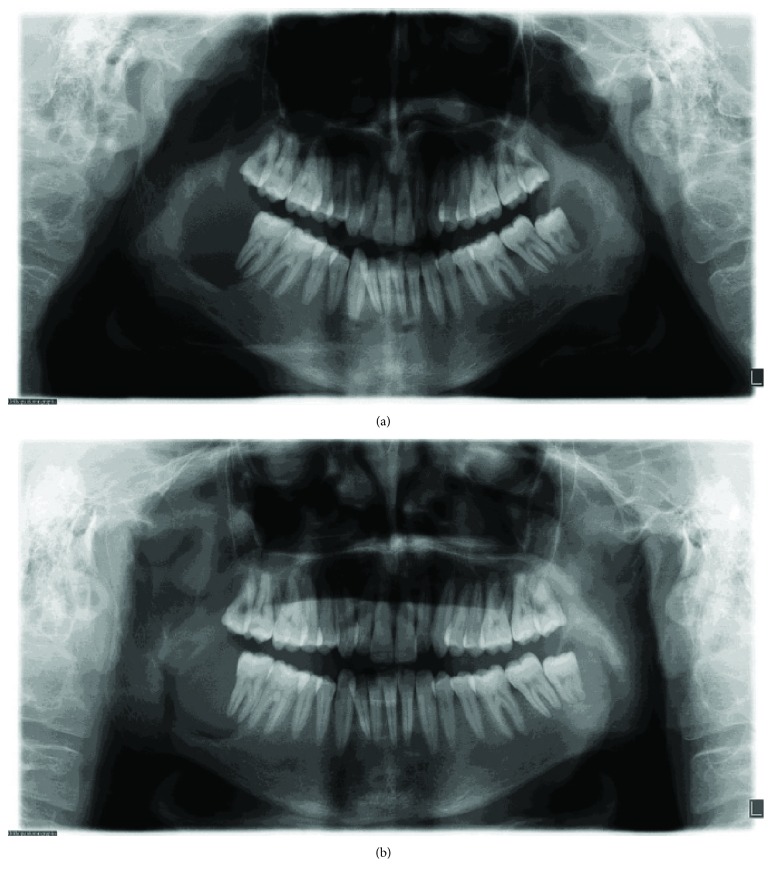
(a) Three months of follow-up: panoramic radiography of bilateral brown tumors. Notice to decreased radiolucency for the brown tumor in the left side of the mandible. (b) First year of follow up: panoramic radiography of bilateral brown tumors. Notice to decreased radiopacity for the brown tumor in the bilateral sides of the mandible.
